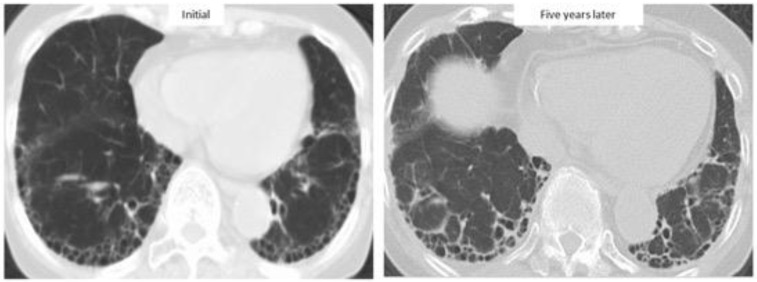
High-resolution computed tomographic images of a patient with a stable course of RA-ILD for five years.
